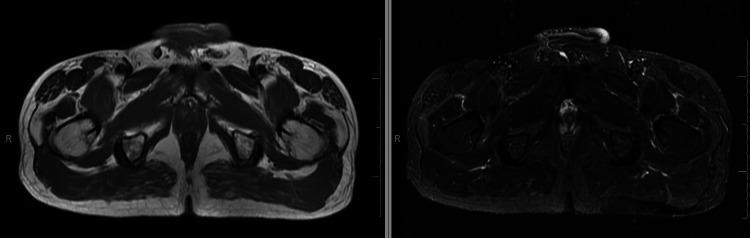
Muscle magnetic resonance imaging of the proximal part of both thighs. Left figure: Axial T1 image. Right figure: STIR image.
